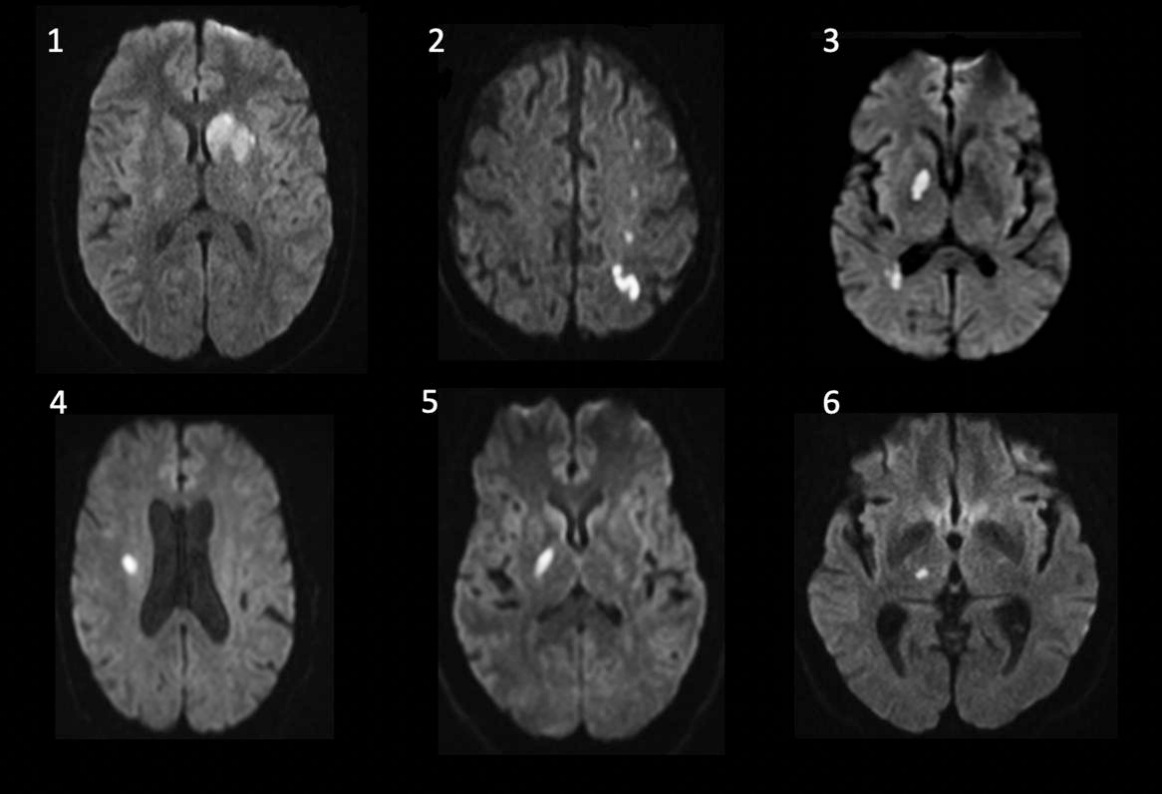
MRI scans. Representative diffusion weighed MRI scans of patients 1–6 (*N* = 6) depicting their small, predominantly subcortical infarcts.
